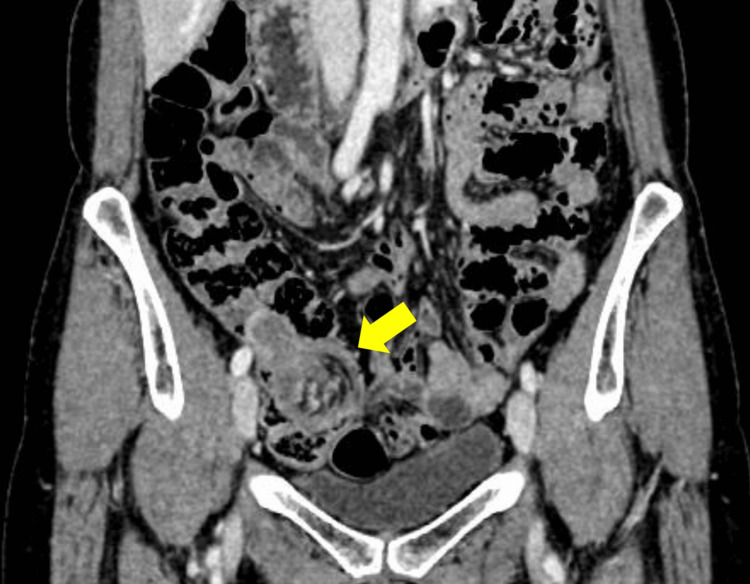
Contrast-enhanced CT (mesenteric vessel converging sign): Mesenteric vessels converging toward the lead mass (arrow) CT: computed tomography
